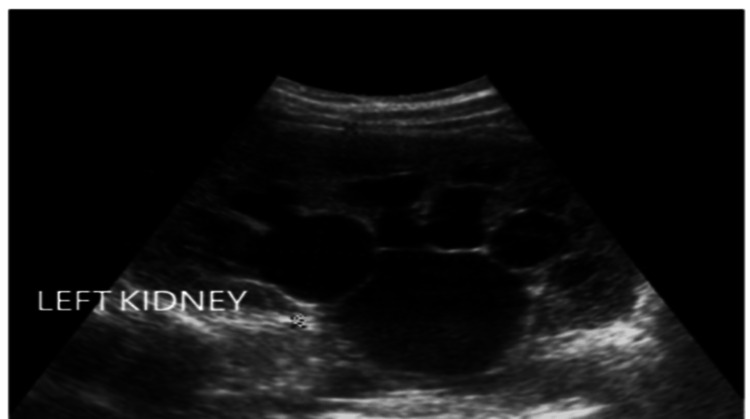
Ultrasound scan at two-three months showing significant left kidney hydronephrosis
